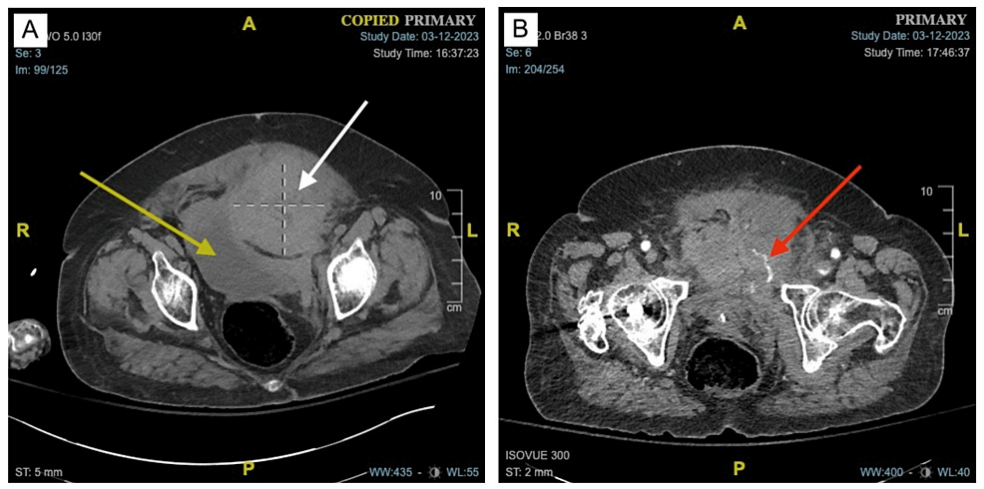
CT showing an anterior pelvic hematoma secondary to a traumatic pelvic fracture. (A) CT without contrast demonstrating a 10 x 9.8 x 9.6 cm extraperitoneal hematoma (white arrow) that is exerting a mass effect on the urinary bladder (yellow arrow). (B) CT angiography showing active internal bleeding into the hematoma (red arrow).
